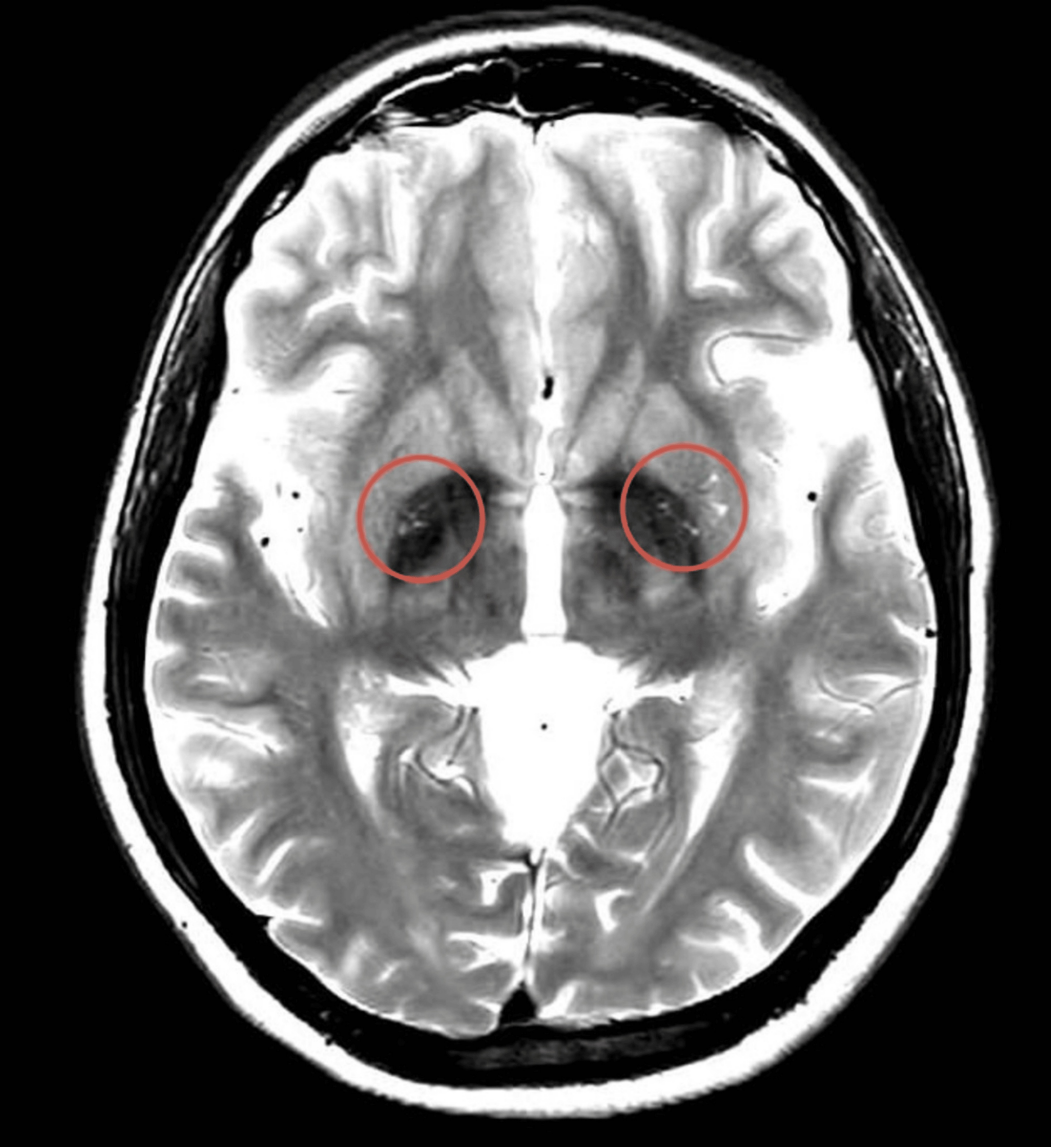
MRI brain showed a few nonspecific white matter hyperintensities
